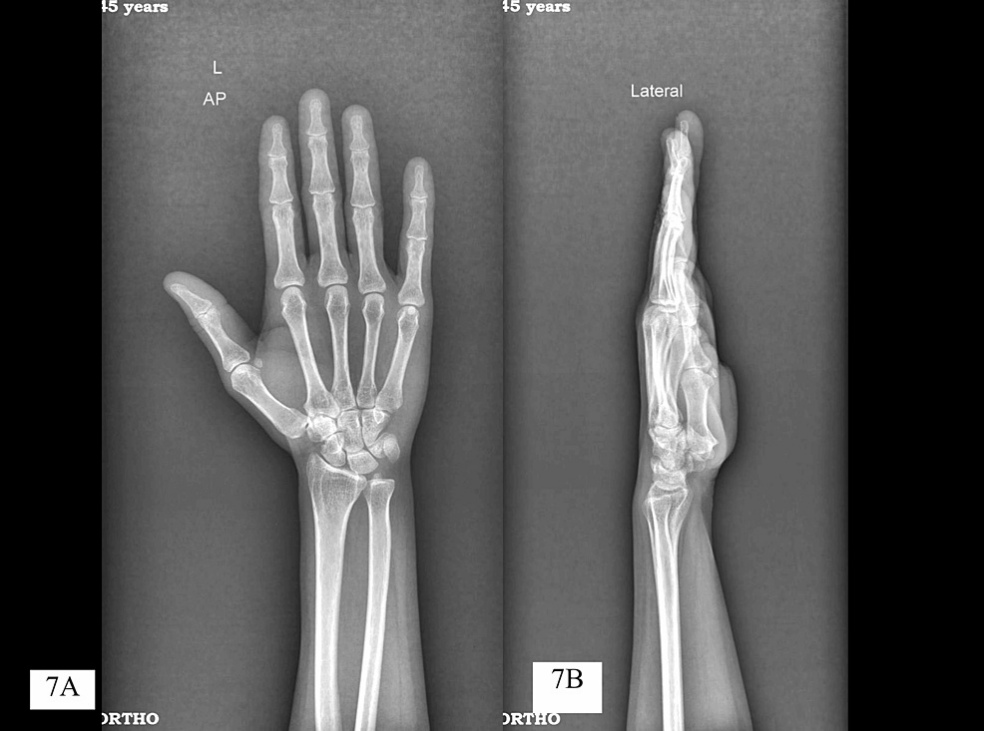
Two-year post-operative X-ray images (A) anteroposterior and (B) lateral view of hand showing no recurrence
